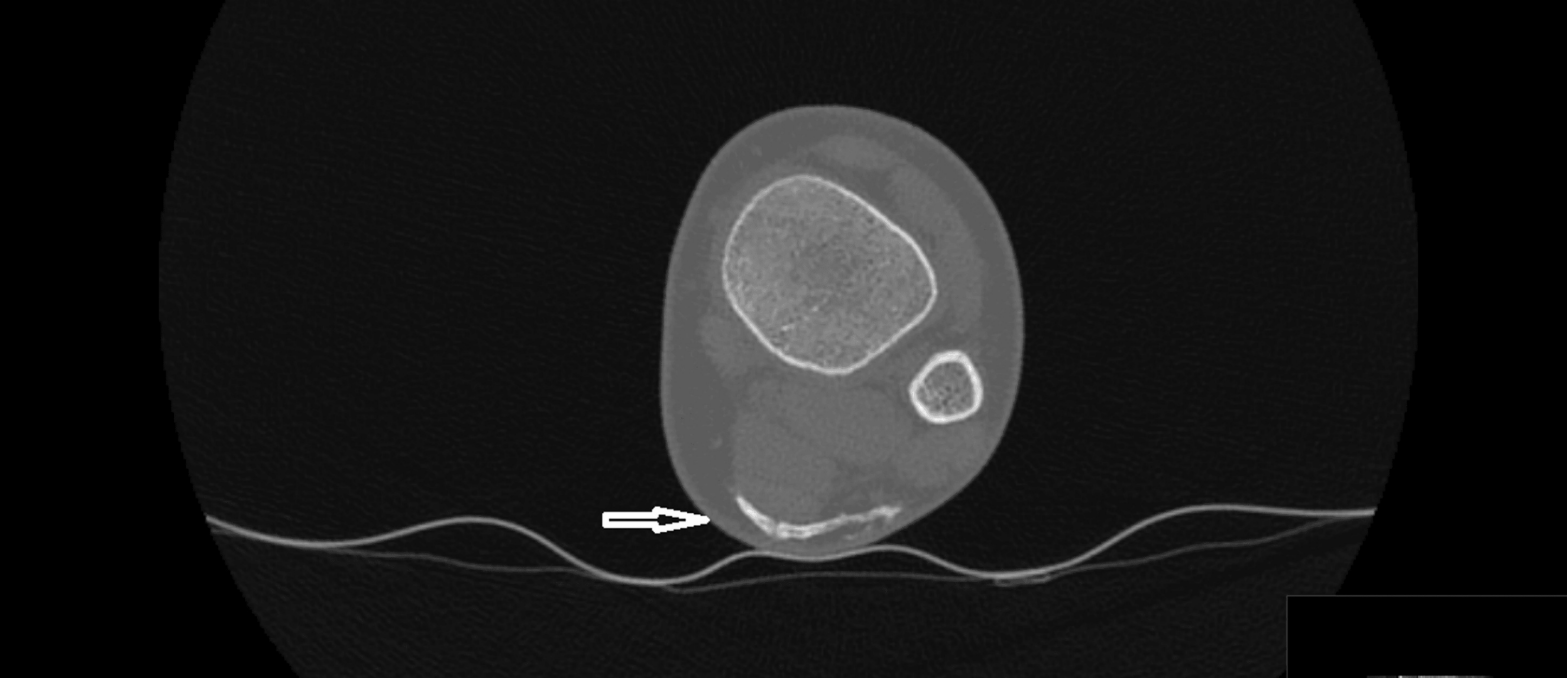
CT image on the axial plane showed a thin calcified lesion, located above the Achilles tendon, extending toward the sural nerve and vessel.
